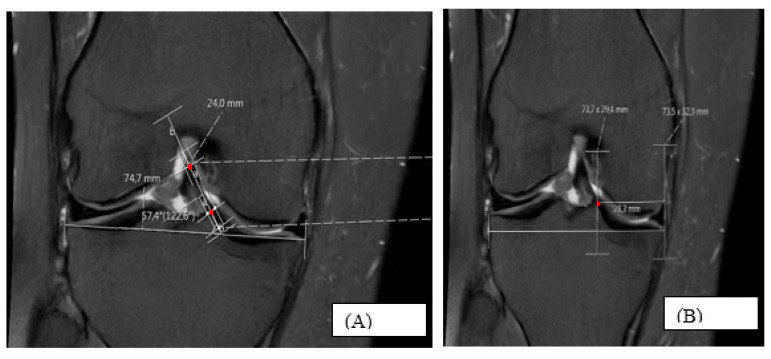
Coronal MRI measurements of the ACL tibial insertion.
